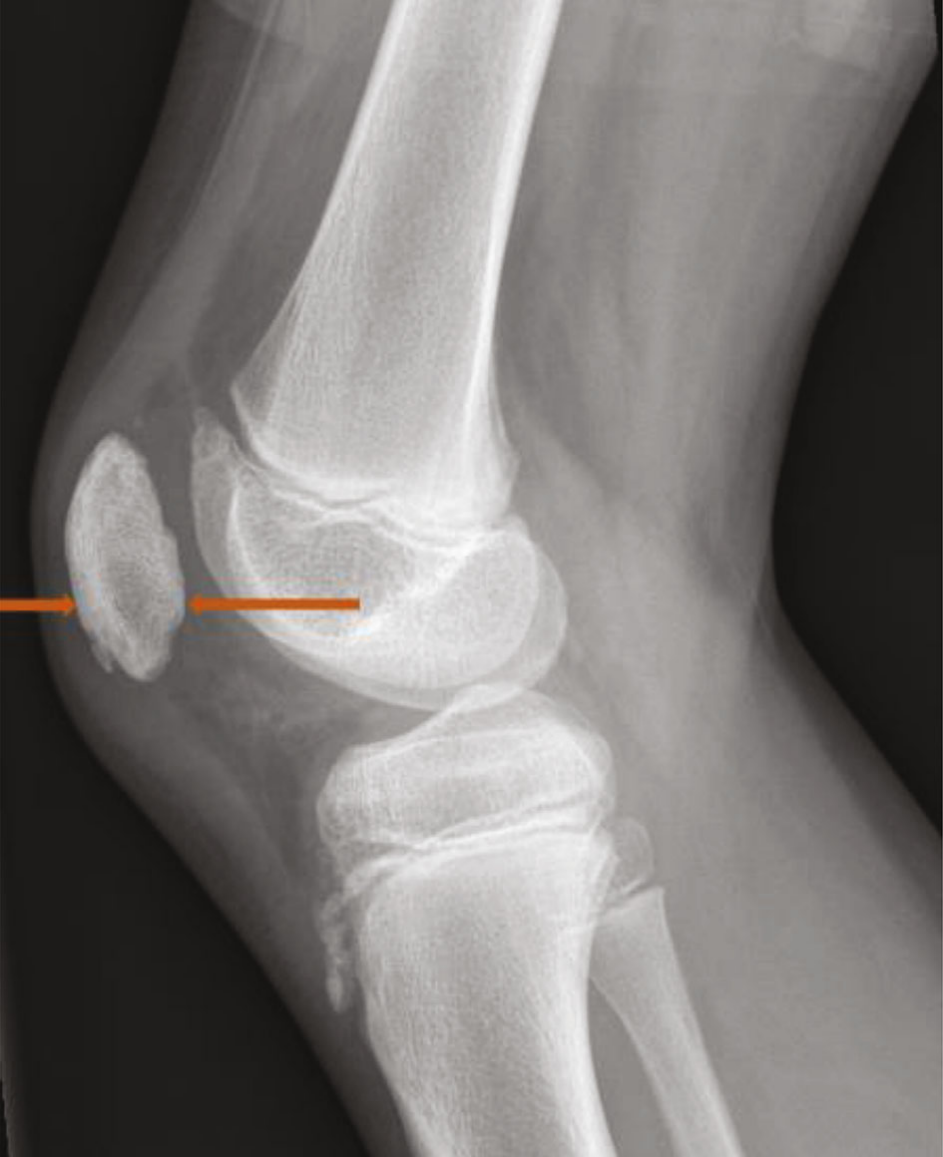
X-ray 4. Lateral view of the right knee, 6 weeks after diagnosis. Orange arrows show the lysis area at the lower pole of the patella.
